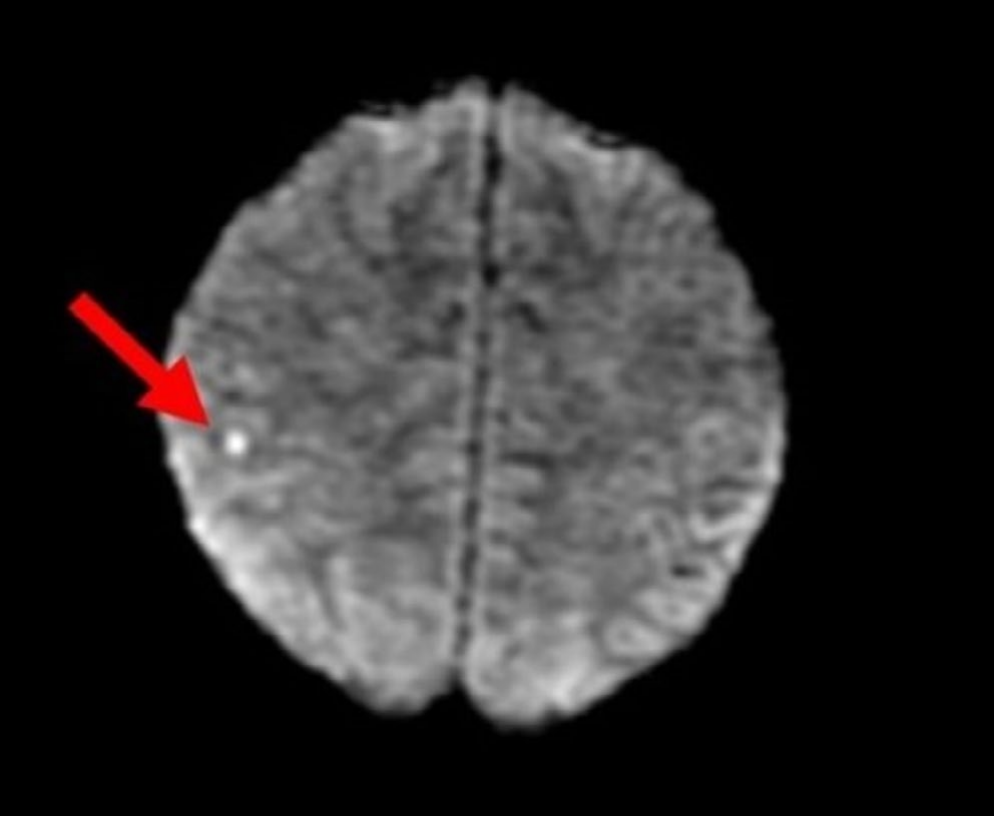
Axial magnetic resonance imaging of the brain showing a 3 mm punctate acute ischemic infarct in the right parietal lobe (arrow).
